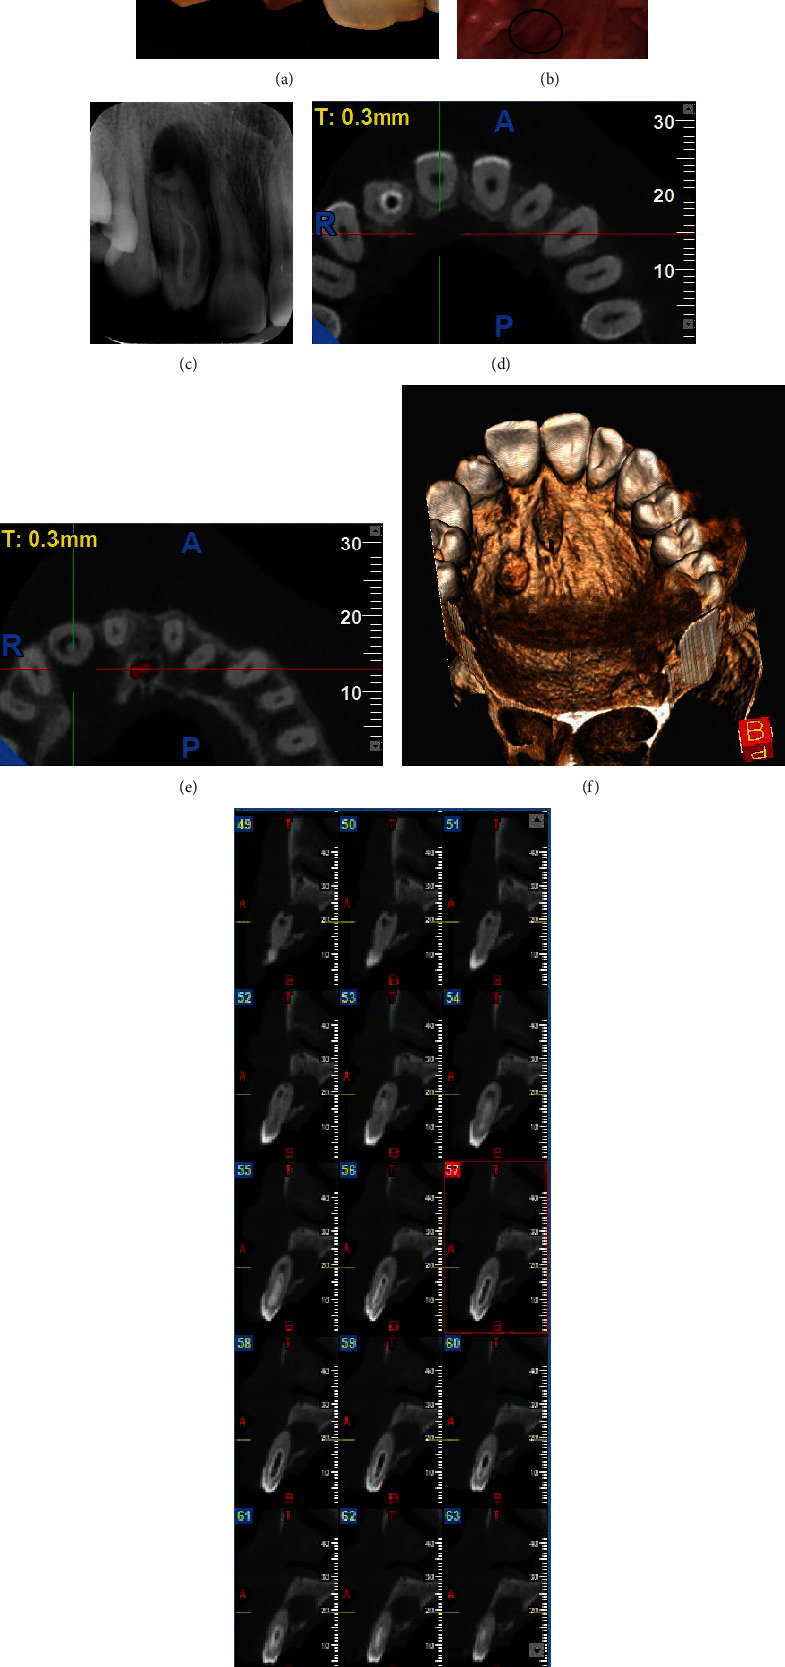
(a, b) Preoperative images of barrel-shaped #7 with palatal nondraining sinus. (c) Periapical radiograph showing complex canal anatomy and periapical lesion. (d–g) CBCT scans showing class 2 dens in dente with #7.
